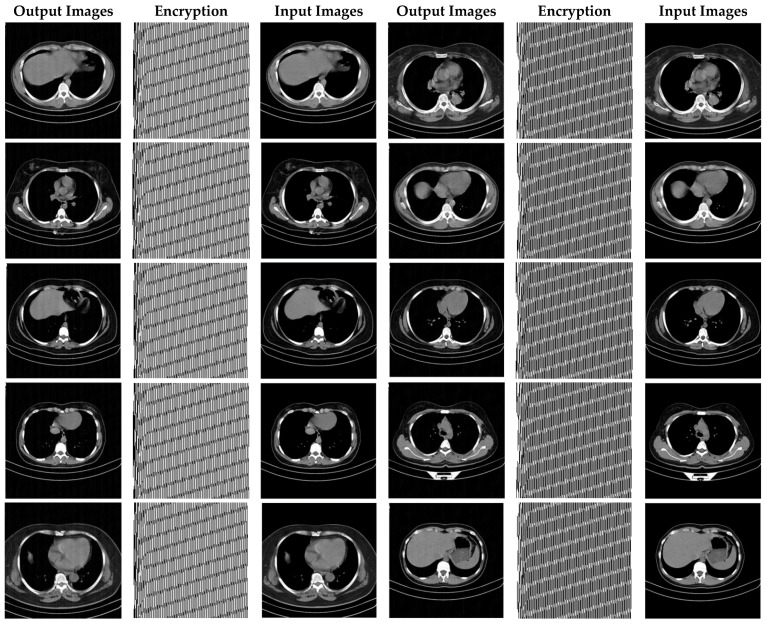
CT images encrypted using the proposed method.
